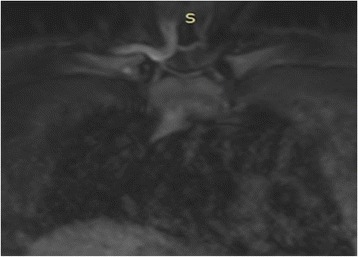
Osteolytic lesion hyperintense on MRI T2 and STIR images
